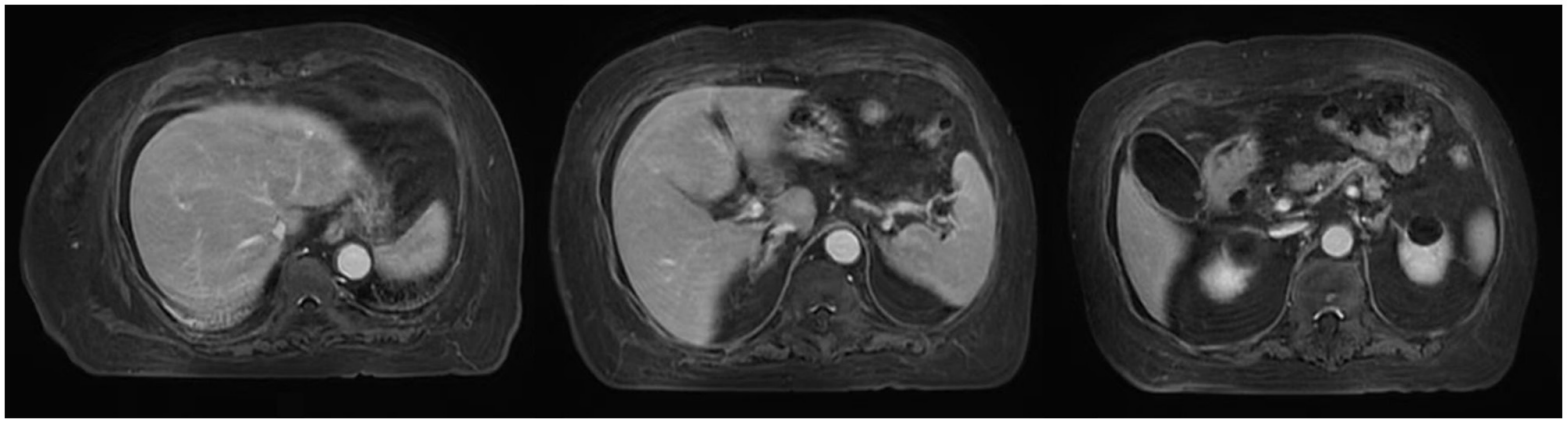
MRI scans in this patient.
